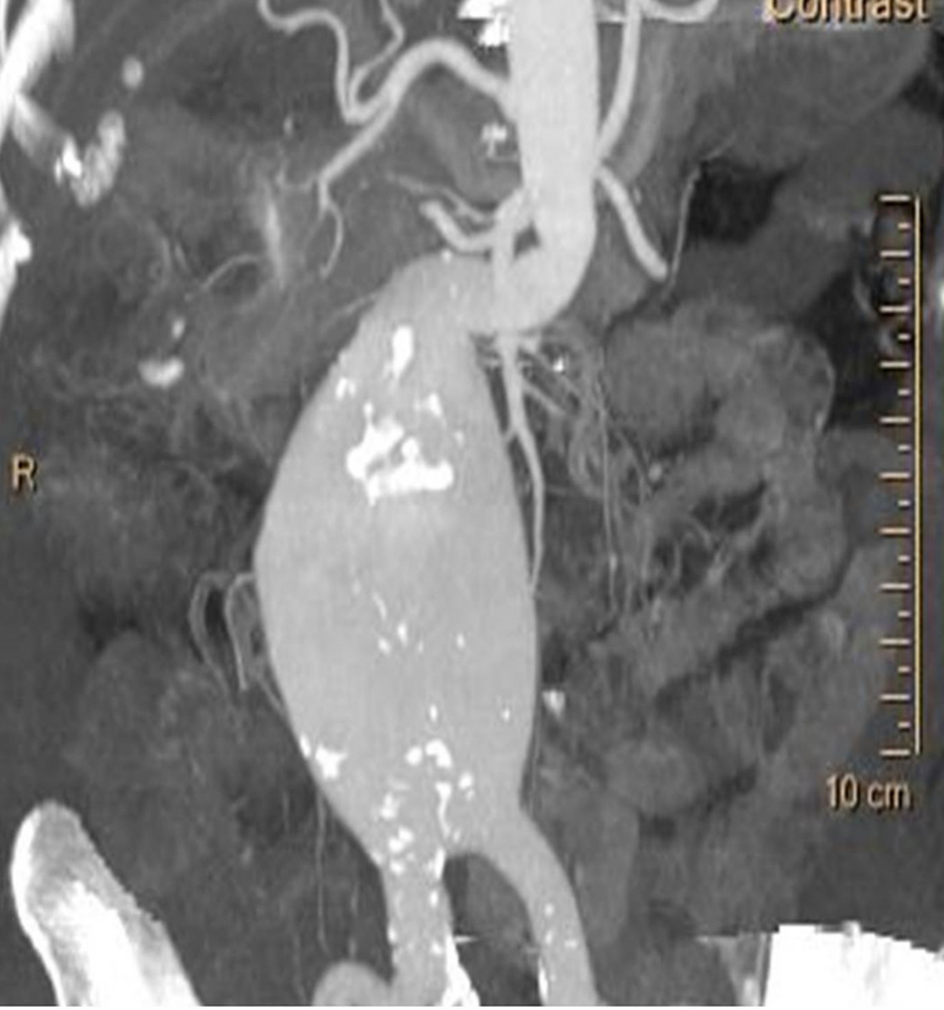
Reconstructed CT angiogram demonstrating the infrarenal abdominal aortic aneurysm and its angulated narrow neck.
